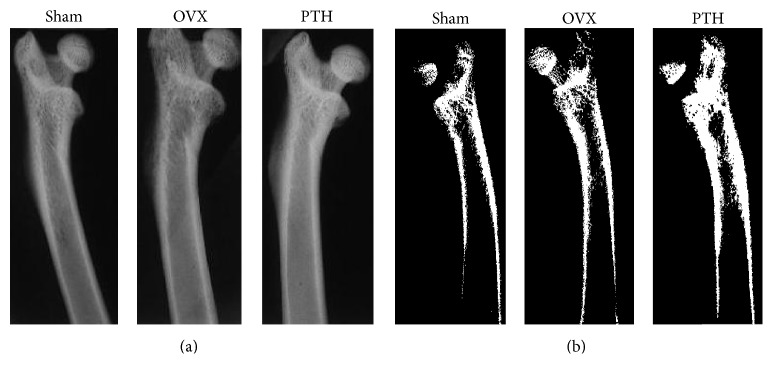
Therapeutic treatment experiment: example of microradiography of Sham control, OVX and OVX treated with PTH femurs bones at 11-weeks postoperation. (a) Representative radiographs (b) Radiographs following threshold analysis. PTH treatment increased the trabecular bone compare with the Sham and the OVX controls. Slides were conducted with FAXITRON (a cabinet X-ray system, Hewlett-Packard).
